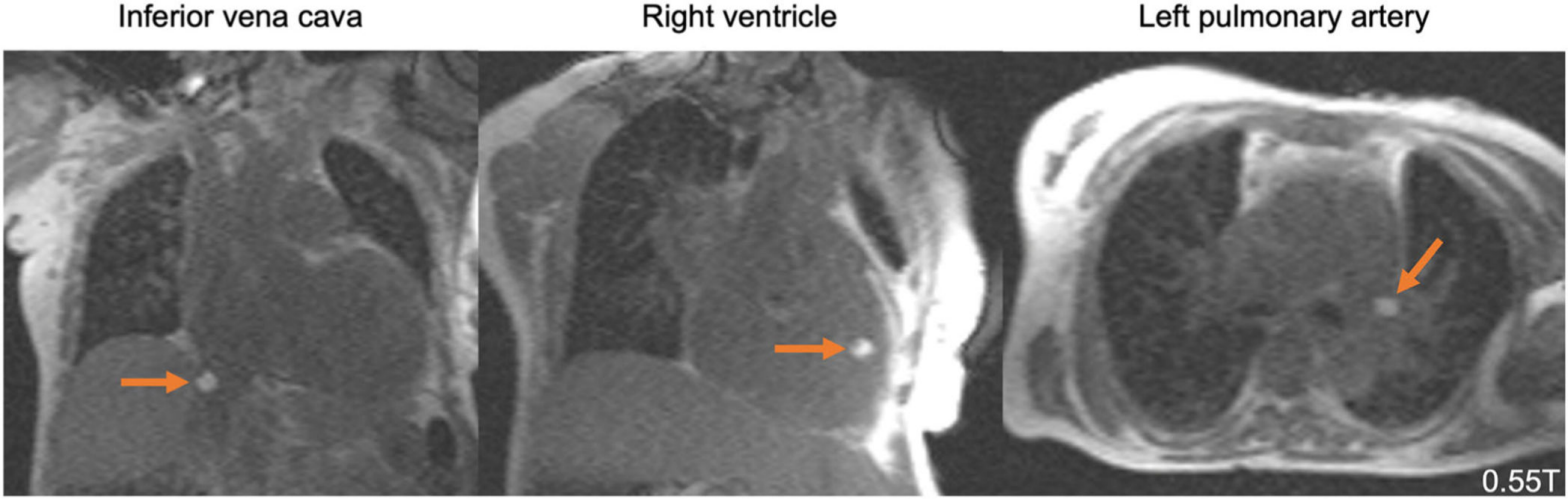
MRI-guided right heart catheterization at 0.55T. Due to device heating, this nitinol guidewire with a gadolinium filled balloon tip catheter could not be used in a 1.5T scanner, but can be safely used for 0.55T MRI-guided procedures. The gadolinium filled balloon is used to navigate the guidewire during right heart catheterization. Images acquisitions are real-time bSSFP with partial saturation pre-pulse (TE = 2 msec, TR = 4 msec, flip angle = 45°, partial saturation pre-pulse flip angle = 60°). Images provided courtesy of Dr. Adrienne Campbell-Washburn, National Heart, Lung, and Blood Institute.
